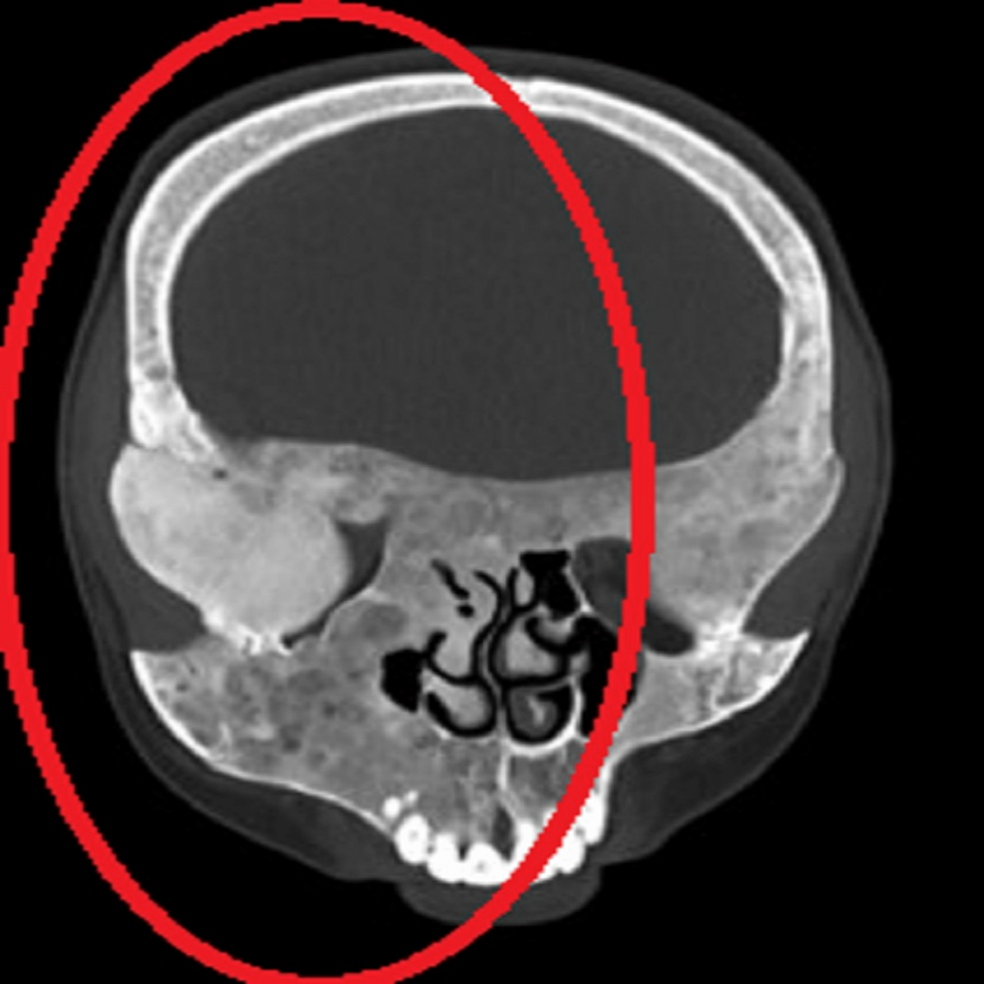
CECT head (coronal cut) showing diffuse ill-defined expansile ground glass lesion involving craniofacial lesion CECT: contrast-enhanced computed tomography
